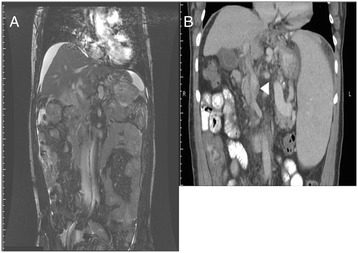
Preoperative imaging study of the abdomen. (A) A magnetic resonance imaging scan showed the relatively small size of the portal vein in both hepatic lobes, which may have been due to the hepatofugal flow or stasis flow causing thrombosis. (B) A computed tomography scan done in the previous hospital revealed the presence of a long segment of portal vein thrombosis (white arrowhead).
